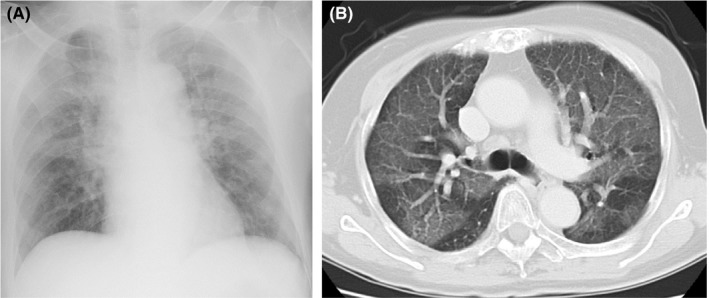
A, B, Chest x‐ray and CT perfomed on admission showed bilateral ground glass opacity dominant on the upper lung field
